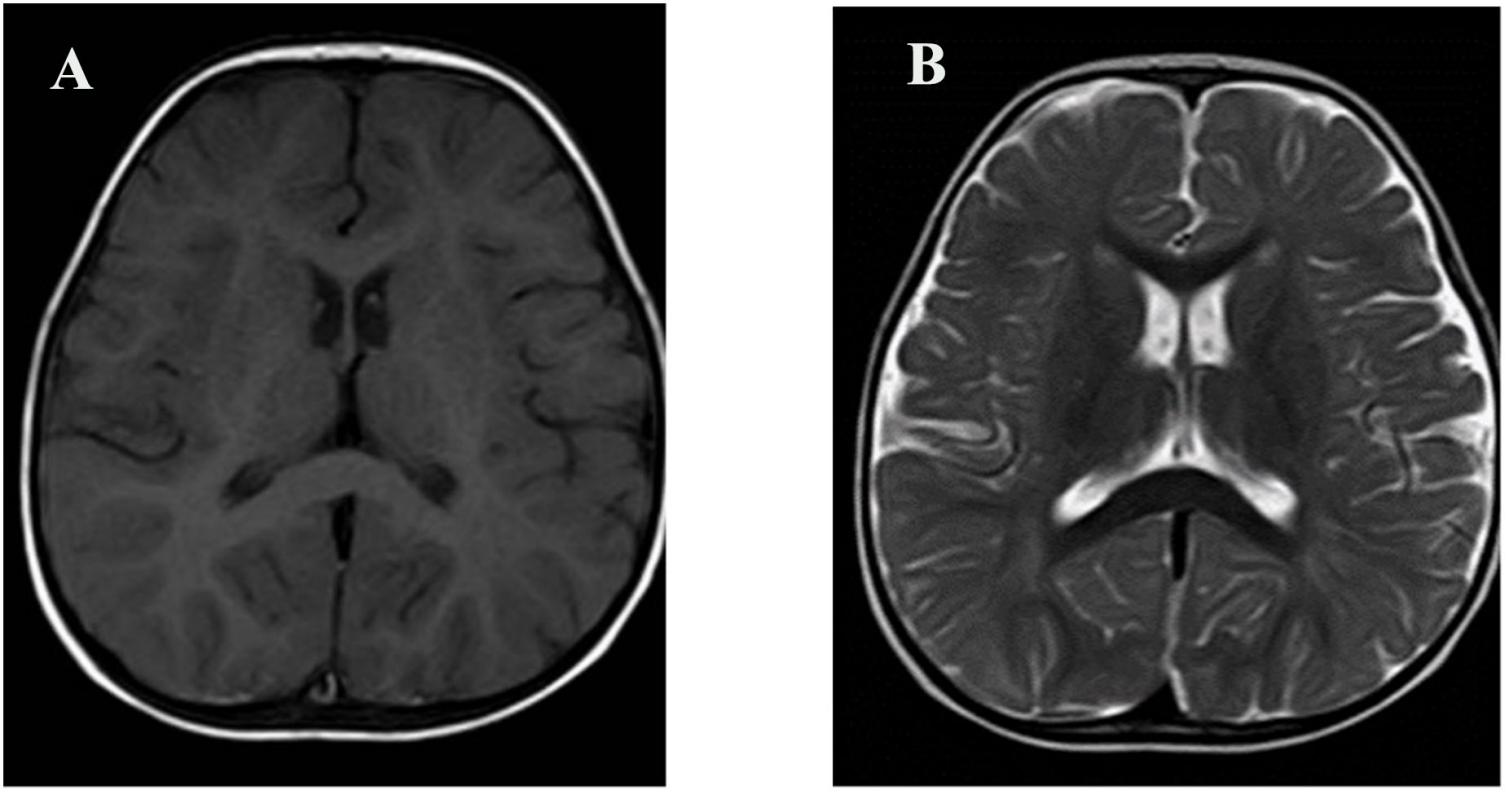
Brain MRI of the patient. (A) Axial T1-weighted image; (B) Axial T2-weighted image. No structural abnormalities were observed.
